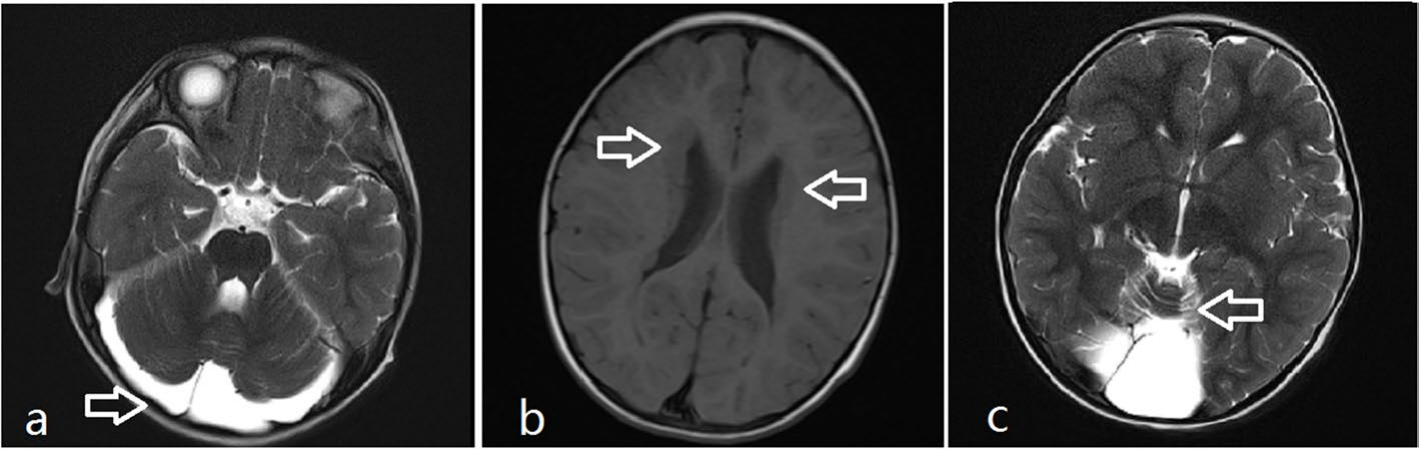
Magnetic resonance imaging (MRI) of the patient suggests multiple nodular gray matter signal shadows in bilateral lateral ventricles, irregular morphology of bilateral lateral ventricles, and significant enlargement of the occipital greater cisterna. The arrow in figure a indicates a large occipital cisterna; The arrows in Figure b indicate multiple nodular gray matter signals in bilateral lateral ventricles; The arrow in figure c indicates hypoplasia of the cerebellum
